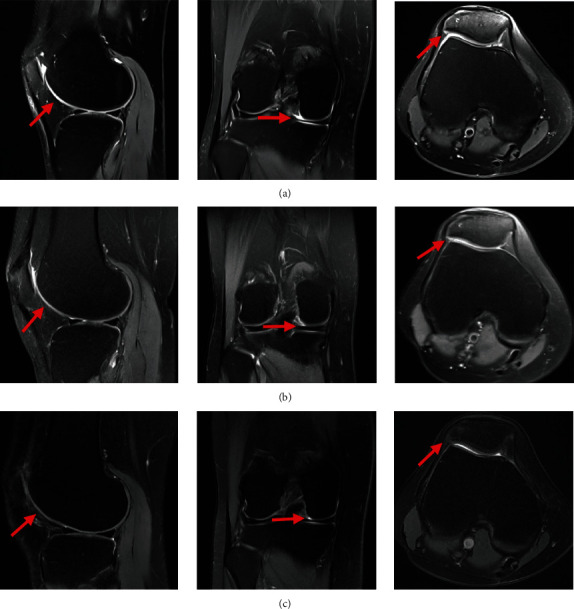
MRI scans of the SVF-treated knees with OA performed at baseline and 6 and 12 months, respectively. It was found that the defect was completely repaired and filled, and the cartilage fused well with adjacent cartilage and subchondral bone in the coronal, transverse, and sagittal planes (red arrow): (a) baseline; (b) 6 months; (c) 12 months.
